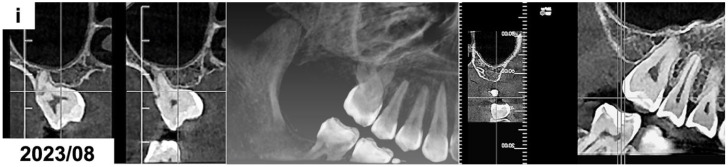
(a) CBCT examination scans before the treatment presenting the odontogenic tumor in the right maxillary molar region. The patient was 15 years and 10 months old. (b) CBCT examination scans after the surgical removal of the tumor presenting the malposition of the impacted permanent upper right first molar. The patient was 16 years and 4 months old. (c) Panoramic radiograph after the treatment, presenting the impacted maxillary. The patient was 16 years and 7 months old. (d,e) Intraoral photographs 10 months after the surgical treatment before the use of orthodontic traction with a segmented fixed appliance. The patient was 16 years and 7 months old. (f) Intraoral photographs 1 year and 4 months after the orthodontic traction of the permanent upper right first molar. The patient was 17 years and 10 months old. (g) Panoramic radiograph 6 years after the surgical and orthodontic treatment. The patient was 24 years and 1 month old. (h) Intraoral photographs 6 years after the surgical and orthodontic treatment showing the erupted permanent upper right first molar and its position in the dental arch. The patient was 24 years and 1 month old. (i) CBCT examination scans 6 years after the surgical and orthodontic treatment presenting the erupted permanent upper right first molar and the absence of the alveolar bone at the site of the tumor removal. The patient was 24 years and 1 month old.
